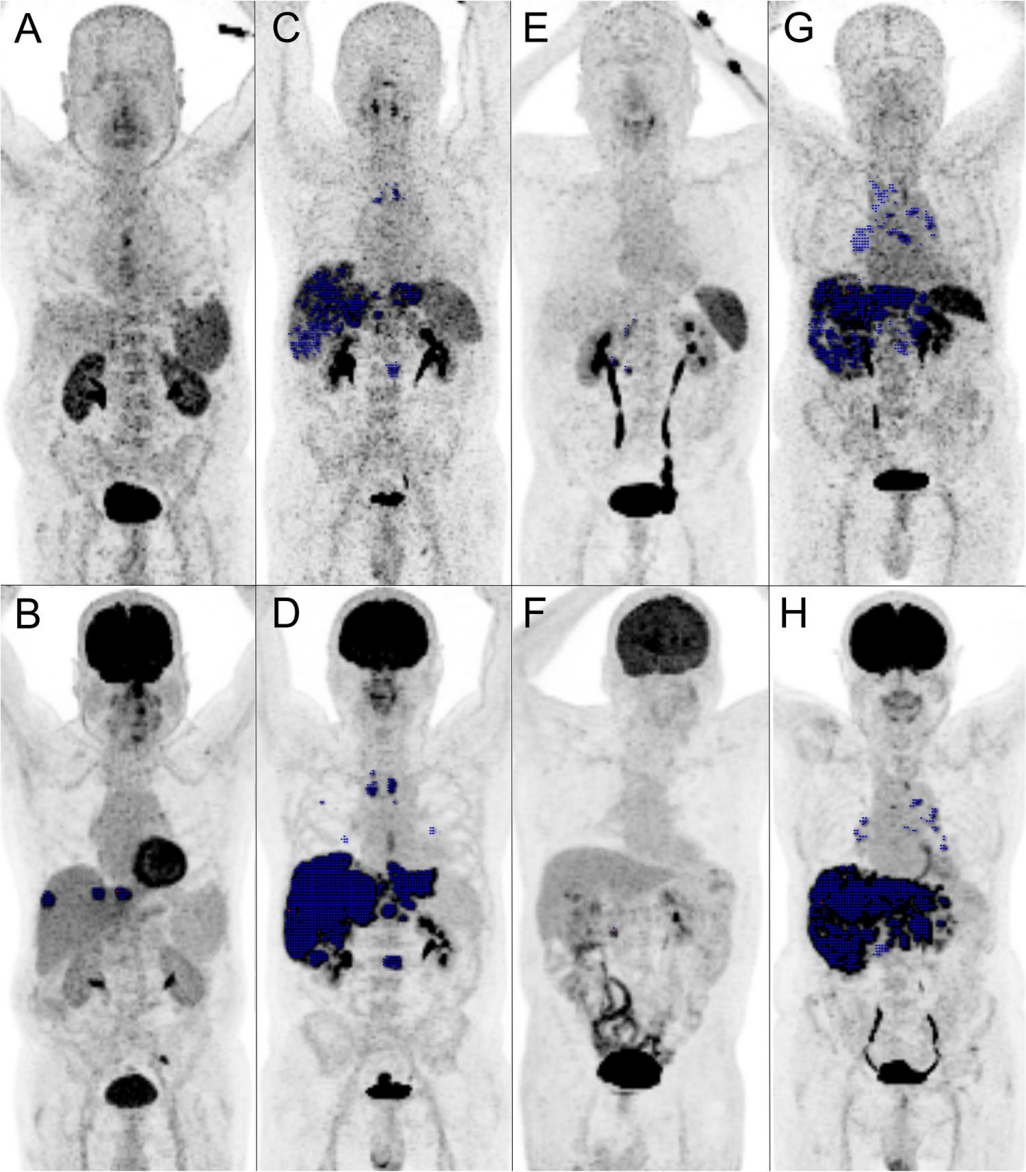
Comparison of PET-based biomarker of corresponding maximum intensity projections of CXCR4 (upper row) and FDG (lower row) PET. Tumor is delineated using Fiji [19] and the Beth Israel Plugin [20] and marked in blue. SUV window ranging from 0 to 5. A, B A 75-year-old patient with pretreated gastric neuroendocrine carcinoma (NEC) and a negative CXCR4 PET scan with missing tracer uptake of the liver metastases. C, D A 55-year old patient with initial diagnosis of an esophageal NEC and metastases in lymph nodes, liver, bones, and the lungs. E, F A 68-year-old patient with initial diagnosis of a pancreatic NEC and local lymph node metastases only seen on CXCR4 PET. G, H A 76-year-old patient with initial diagnosis of a gastric NEC and metastases in lymph nodes, liver, bones, and the lungs
